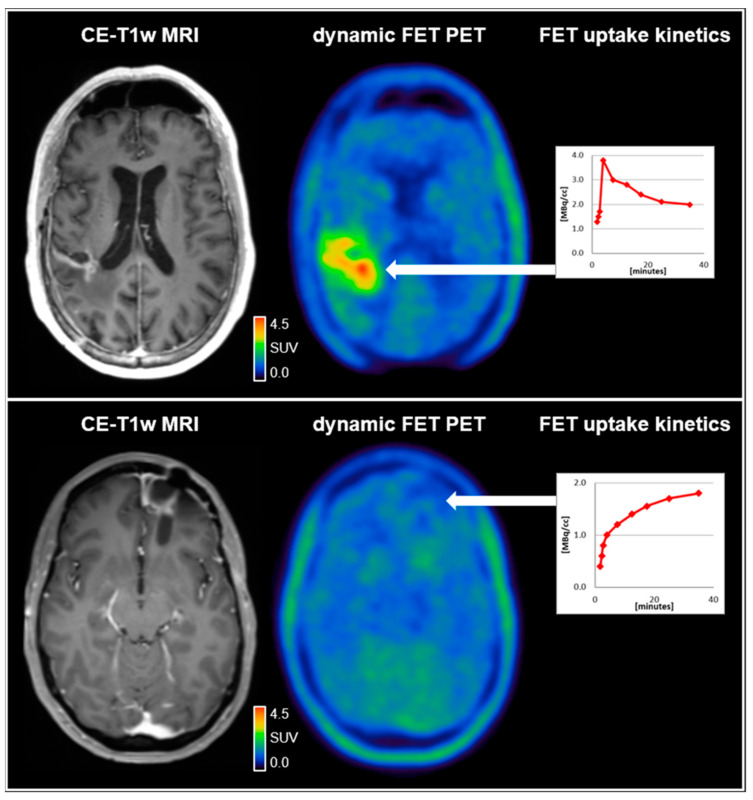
(upper row) A 59-year-old male patient diagnosed with an IDH-wild-type glioblastoma (WHO CNS grade 4). Following resection and chemoradiation with temozolomide, the contrast-enhanced MRI (CE-T1w MRI) suggested tumor relapse in the right parietal region 7 months after completing radiotherapy. Accordingly, the dynamic FET PET scan revealed pathologically increased FET uptake right parietal (TBR_max_, 4.2) and decreased time–activity curve; (lower row). A 37-year-old female patient diagnosed with an IDH-wild-type glioblastoma (WHO CNS grade 4). Following resection and chemoradiation with temozolomide, the contrast-enhanced MRI suggested tumor relapse in the left frontal region 7 months after completing radiotherapy. In contrast to the patient in the upper row, the FET uptake in the left frontal region was not pathologically increased (TBR_max_, 1.6) with a steadily increasing time–activity curve, indicating reactive treatment-related changes. SUV = standardized uptake value.
